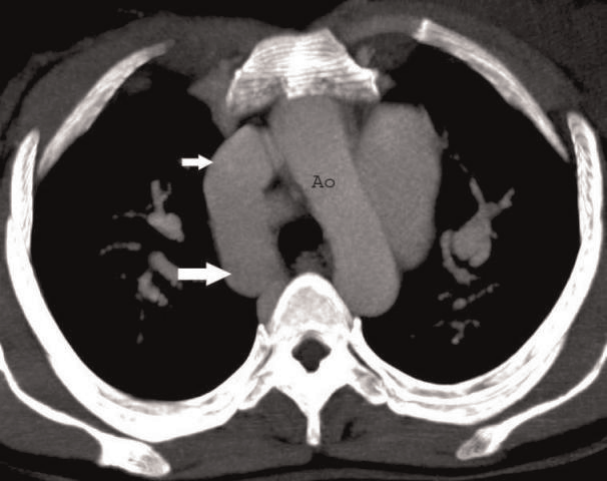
Axial CT image demonstrates vena cava superior (white small arrow) and enlarged azygos vein (white large arrow). (Ao: Aorta).
